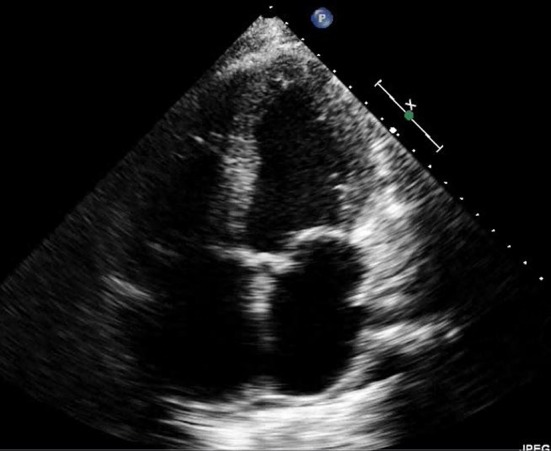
Transthoracic echocardiography (in end-systolic phase) shows an evidence of severe cardiomyopathy with ejection fraction < 20%.
